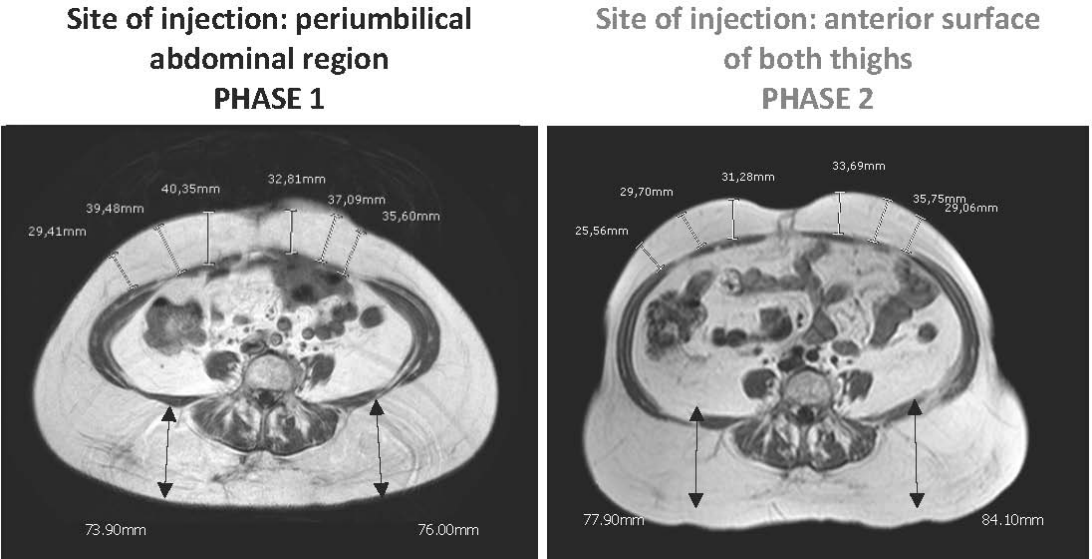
Abdominal MRI scans of patient 2 when injecting pegvisomant (10 mg/day) subcutaneously in her abdomen (Phase 1) and thighs (Phase 2).
